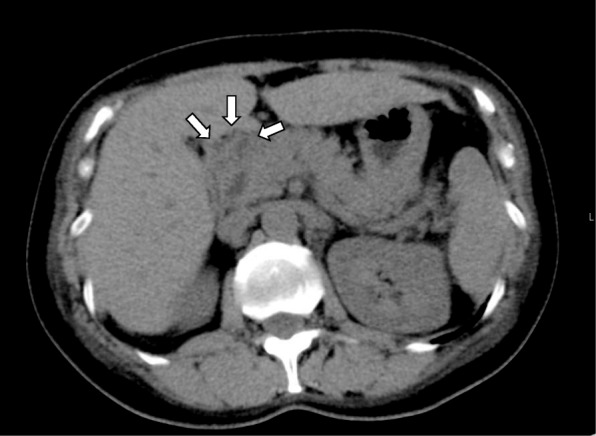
The CT image taken on Day 23 showing edematous changes around the head of pancreas (denoted by arrows). The finding is compatible with a diagnosis of acute pancreatitis
